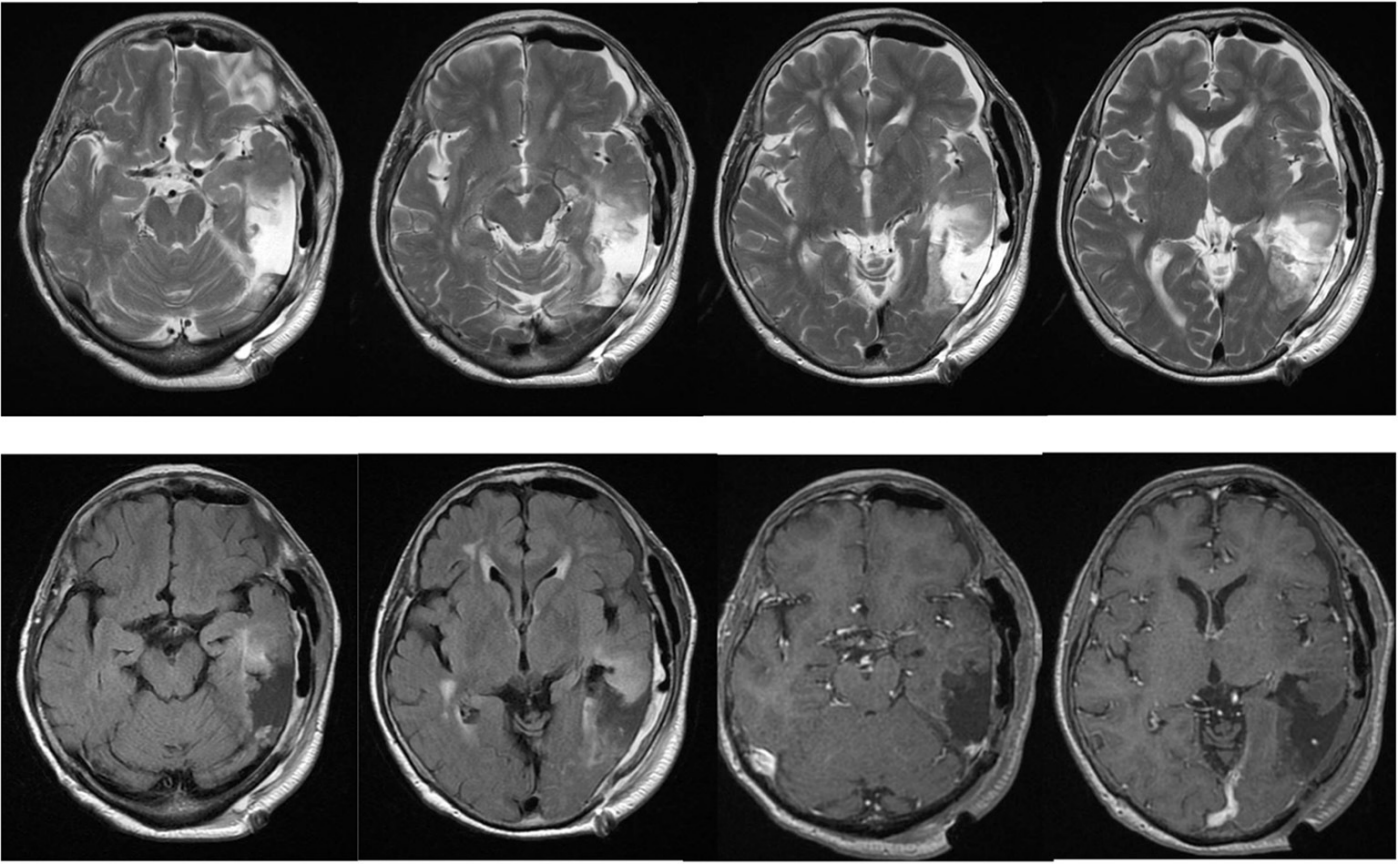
Postoperative MRI showing gross total removal of the tumor.
